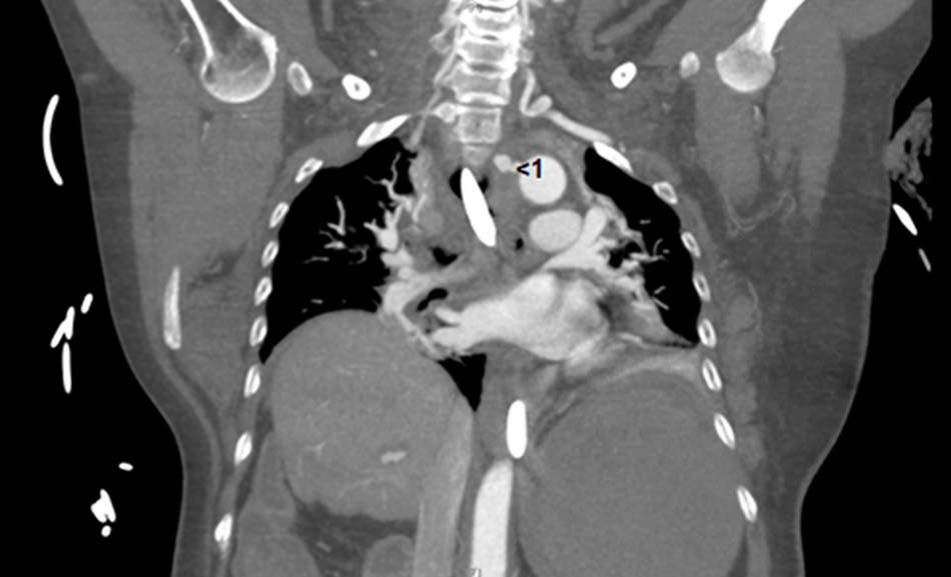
Coronal CT angiograph. This image demonstrates the unusual arterial fistula (red number 1) connecting the arch of the aorta to the oesophagus.
